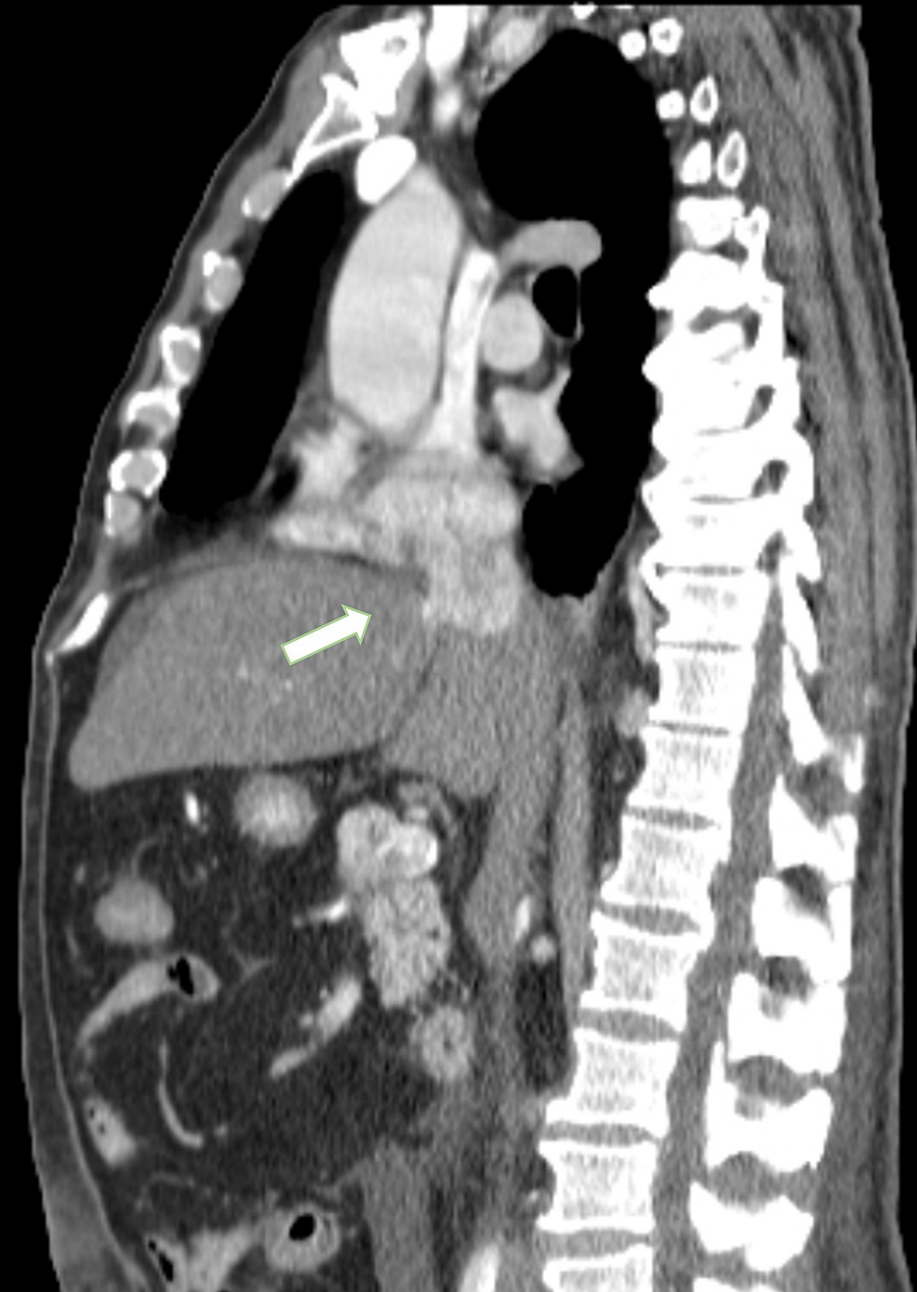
Computerized tomography (CT) with angiography with right atrium invasion Computerized tomography (CT) with angiography showing contiguous right atrium invasion (arrow).
